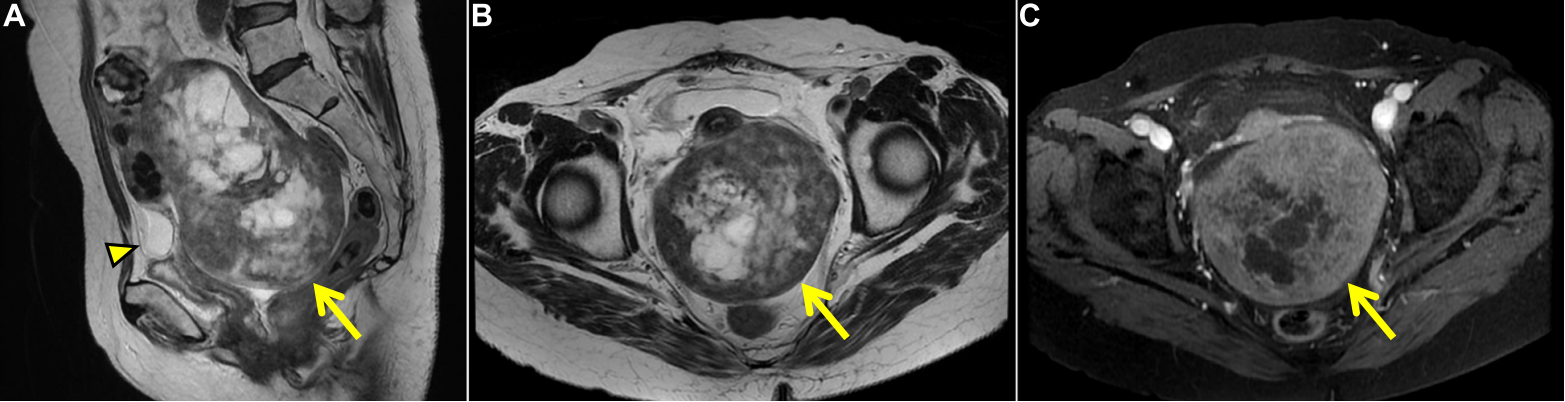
Pelvic magnetic resonance imaging (MRI). a Sagittal and b axial T2-weighted, and c axial gadolinium-enhanced fat-suppressed T1-weighted images. MRI shows the right huge ovarian mass in 14-cm of the long axis, with a multilocular cystic component in the central tumor, and demonstrates the peripheral solid portion as an obvious hypointense area on T2-weighted image and slight and inhomogeneous gadolinium enhancement (arrows). A trivial ascites in the vesico-uterine pouch is also observed (arrowhead)
